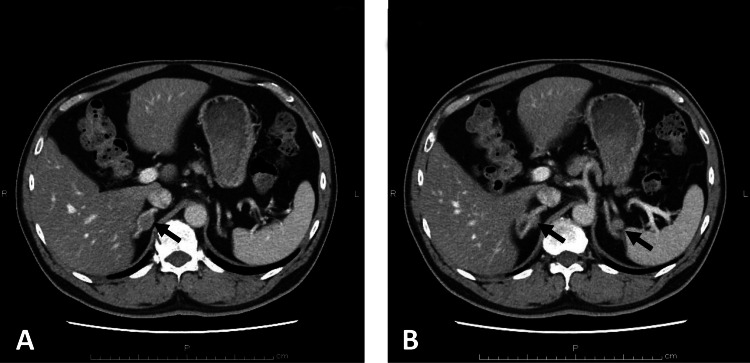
Contrast-enhanced computed tomography at initial presentation demonstrating The figure shows bilateral adrenal nodules (A, 19 mm; B, 12 mm, arrows) with relatively well-defined margins and slightly reduced enhancement compared with the surrounding adrenal tissue.
